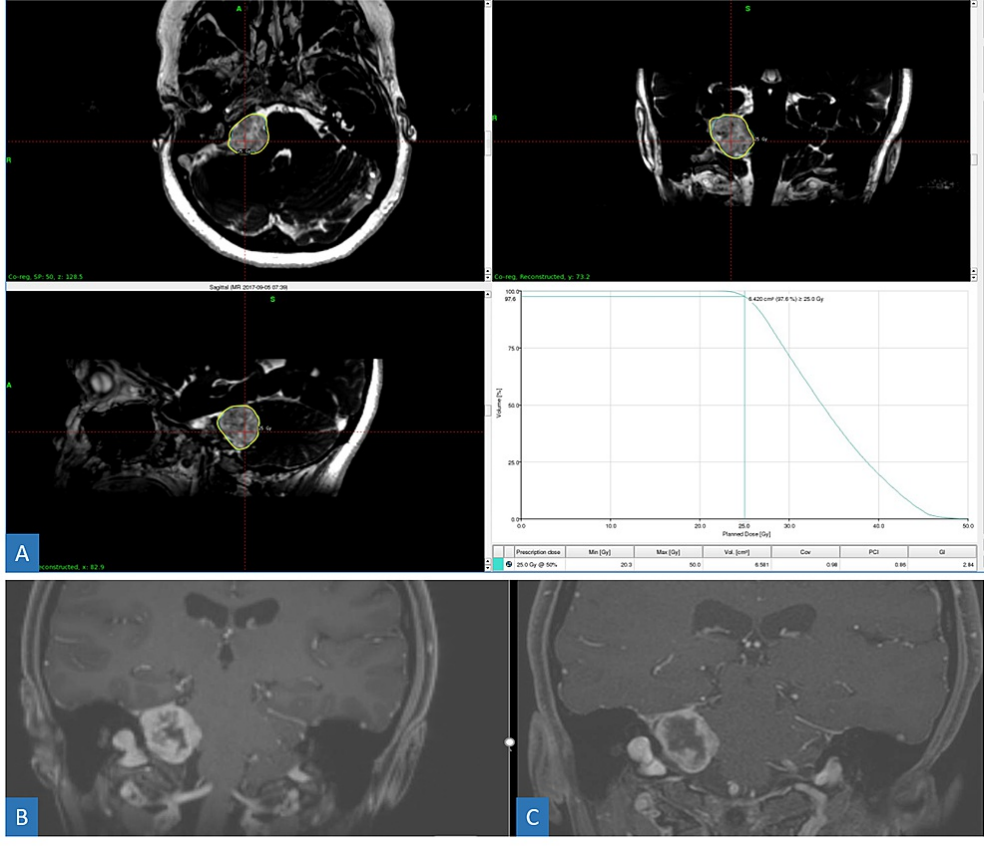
Patient with vestibular schwannoma. Treatment plan (A) for a right-sided vestibular schwannoma which received frameless treatments of 25 Gy in five fractions on the GK Icon. Pre-treatment (B) and post-treatment (C) coronal T1 post-contrast MRI of a right-sided acoustic neuroma. Notice the dramatic increase in central necrosis post-treatment, characteristic of an excellent treatment response. GK = Gamma Knife; MRI = magnetic resonance imaging
